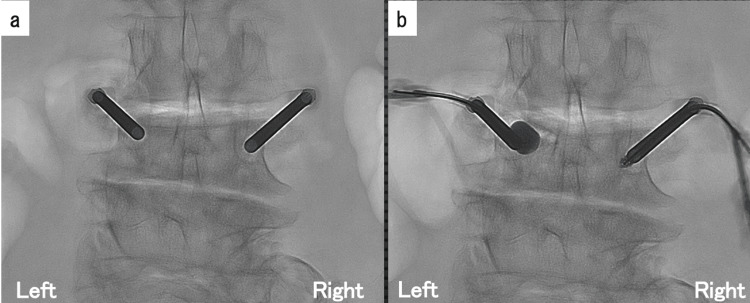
Balloon kyphoplasty (BKP) a) Before balloon kyphoplasty (BKP), X-ray showed the left side vertebral height loss; b) After BKP, X-ray revealed corrected vertebral body height.
